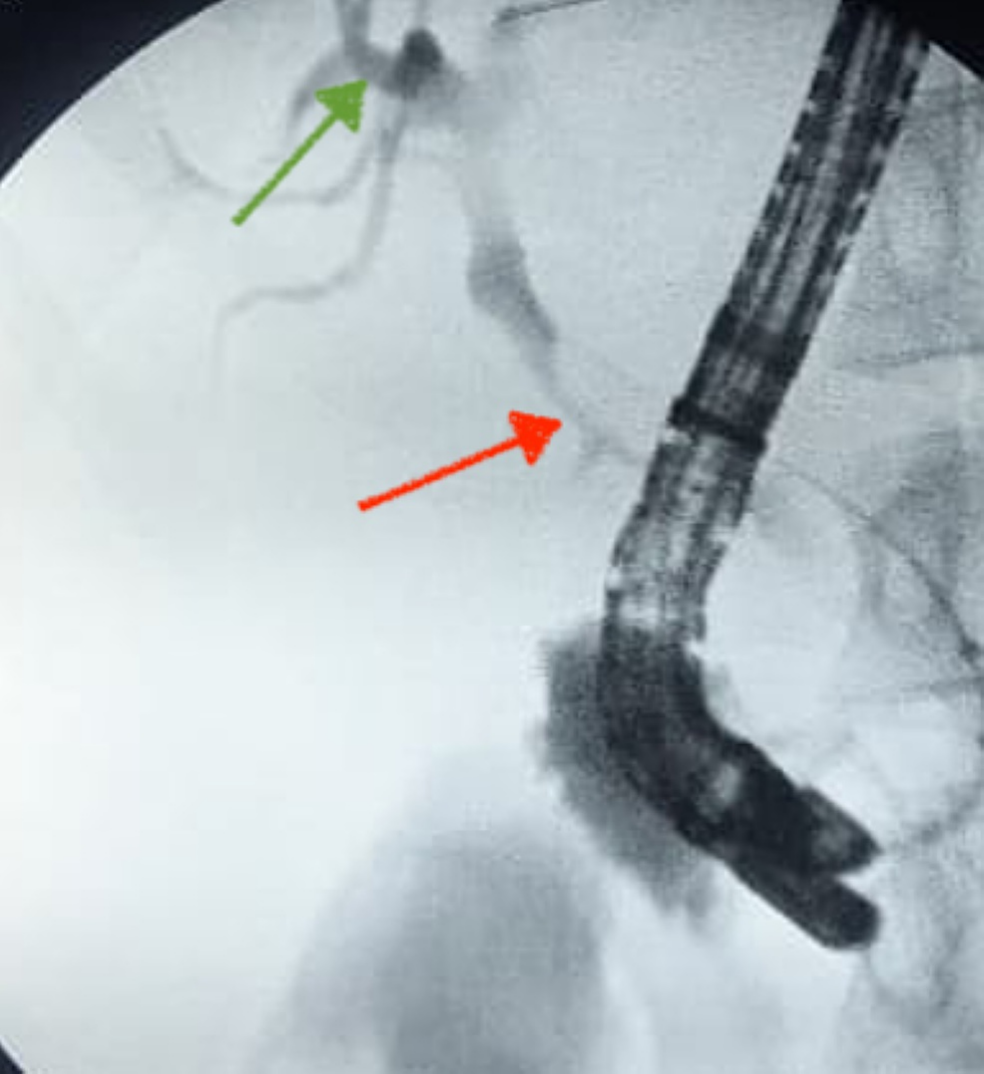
Endoscopic retrograde cholangiopancreatography: X-ray image after opacification showing stenosis of the main bile duct (red arrow) with dilation of the intrahepatic bile ducts (green arrow).
